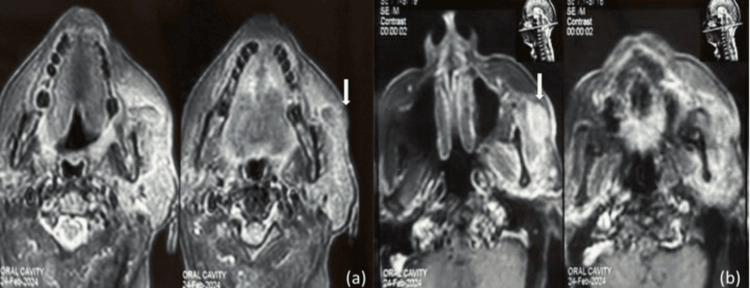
(a) T2W MRI axial section showing heterogeneous iso to hyperintense tumor with loss of subcutaneous fat plane (white block arrow); (b) T1W gadolinium MRI axial section showing hyperintense tumor (white block arrow)
